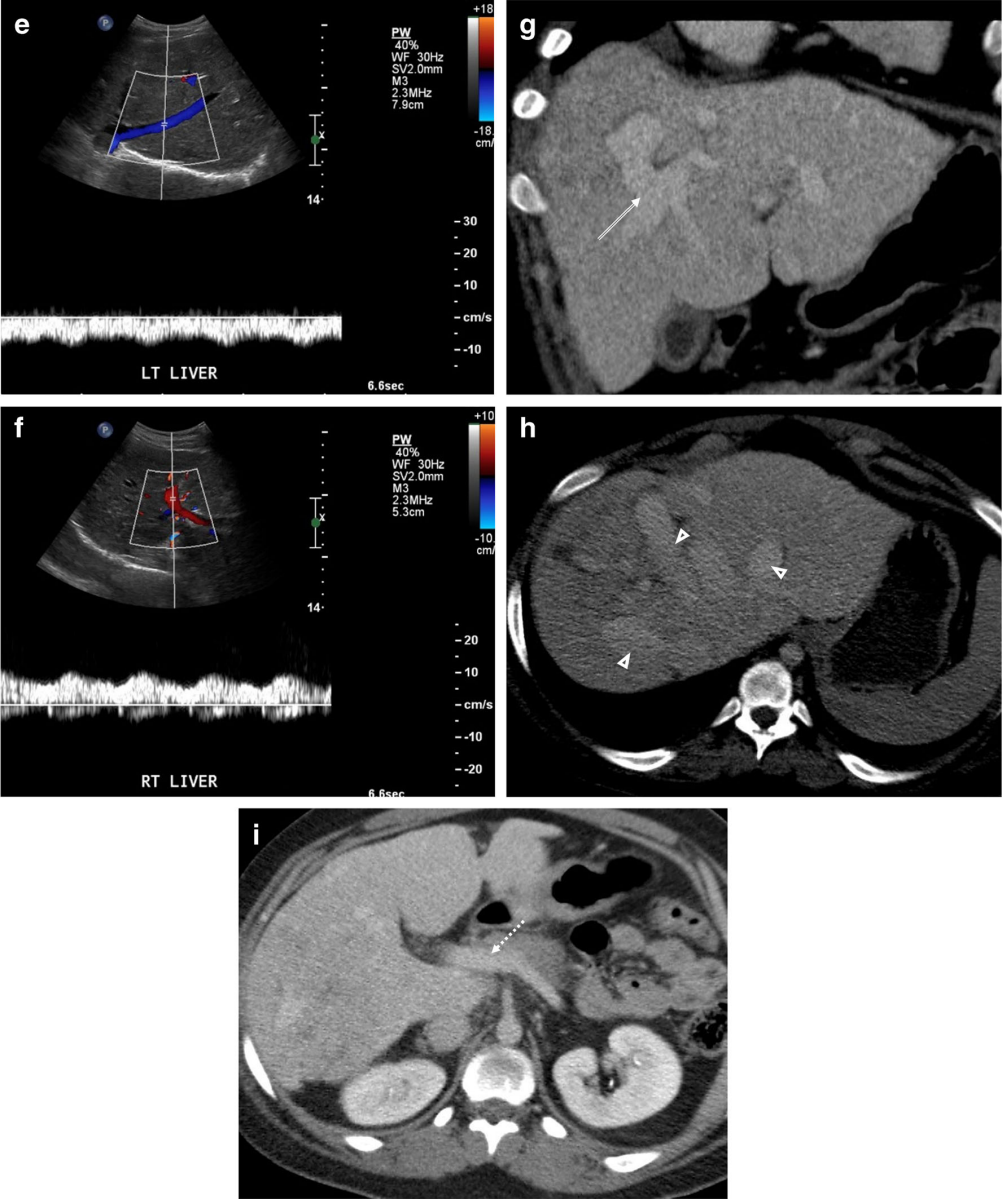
Vessel evaluation in post-Fontan patients. a-c A 31-year-old woman with previous pulmonary atresia with intact ventricular septum. a Coronal CT shows indirect diversion of both SVC and IVC to pulmonary arteries via the right atrium (*asterisk*), corresponding with atriopulmonary anastomosis. b, c Spectral Doppler US examination. b Middle hepatic vein shows preservation of hepatopetal blood flow (*arrows*). c Right portal vein shows heterogeneously coarse hepatopetal flow. d-f A 21-year-old woman with previous left atrial isomerism with pulmonary atresia. d Coronal CT shows direct diversion of both the SVC and IVC to pulmonary arteries, corresponding with total cavopulmonary anastomosis. Situs inversus is also noted. e, f Spectral Doppler US examination. e Left-side hepatic vein demonstrates disappearance of hepatopetal blood flow. f Right-side portal vein demonstrates relatively homogeneous hepatopetal flow. g-i A 33-year-old woman with previous hypoplastic right ventricle with tricuspid atresia. Coronal (g) and axial (h, i) CT images in the portal venous phases demonstrate intrahepatic venous-venous collateral formation (*double-lined arrow* in g), enlarged hepatic veins (*arrowheads* in h), and small main portal vein (*dotted arrow* in i)
